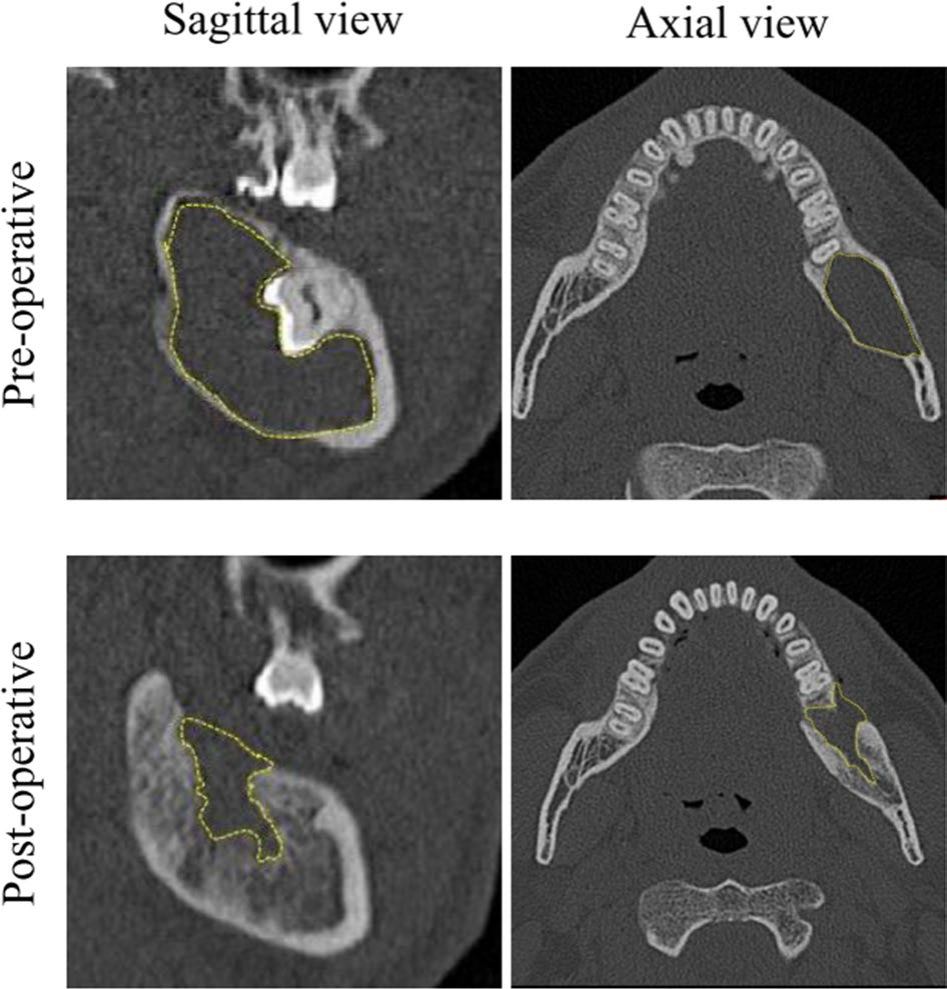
3D measurement protocol by using computed tomography. After a ROI was delineated in one section, the border of the defects (yellow dotted line) was automatically generated for each slice on the axial and sagittal views, respectively. The defect volume was calculated through 3D reconstruction.
